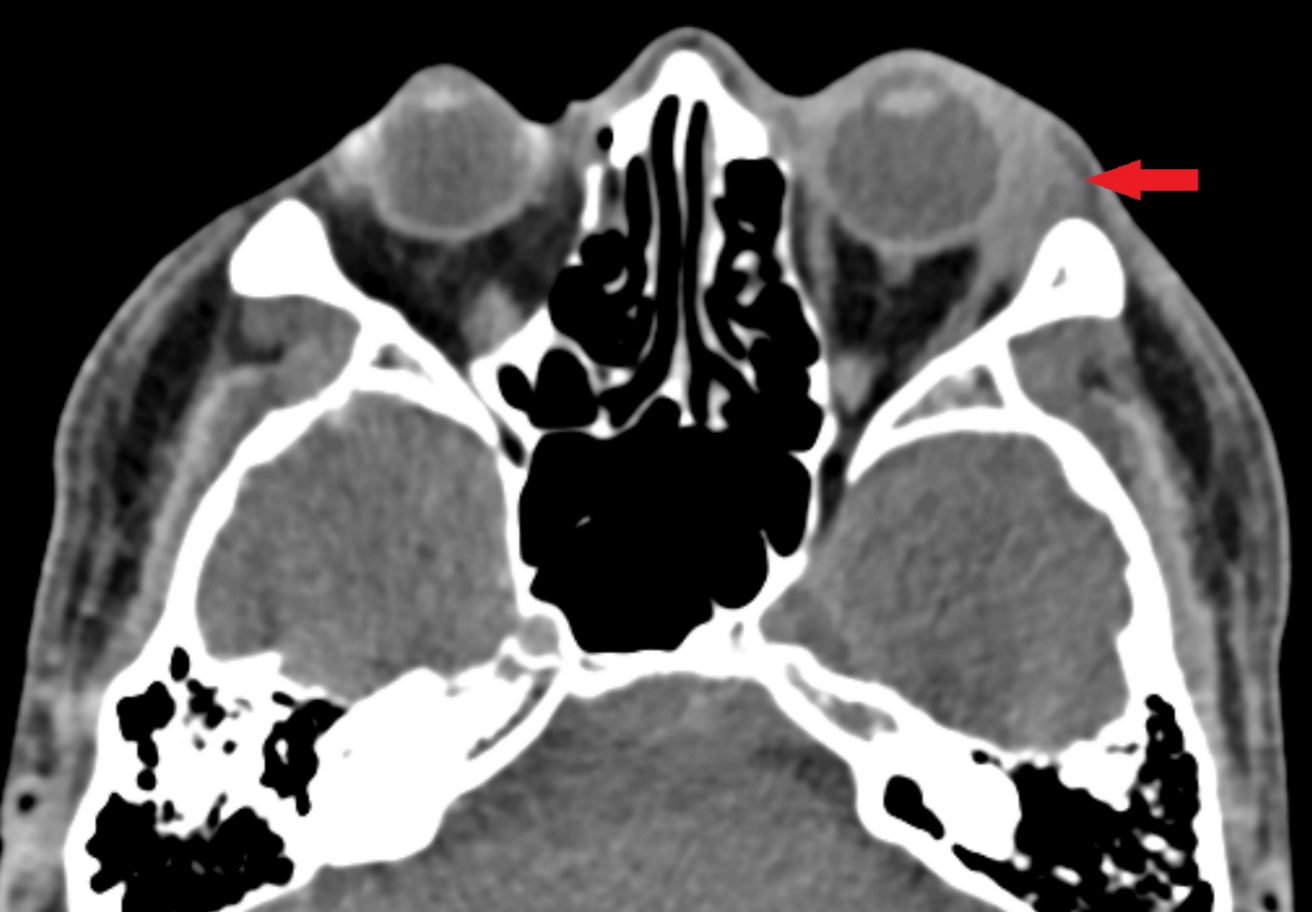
CT orbit showing enlarged left lacrimal gland (arrow).
